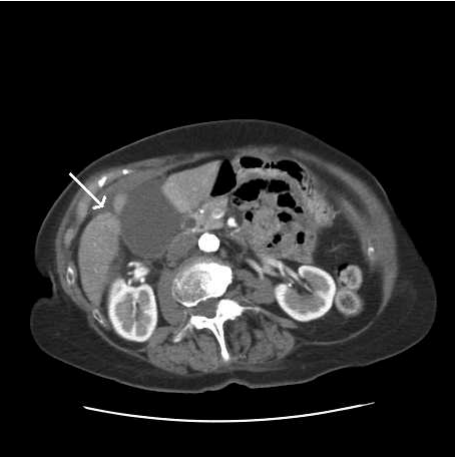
Abdominal computed tomography scan. Focal thickening of gallbladder neck, a hugely distended and inflamed gallbladder as well as fluid in the anterior hepatic space (as indicated by the arrow) can be seen.
